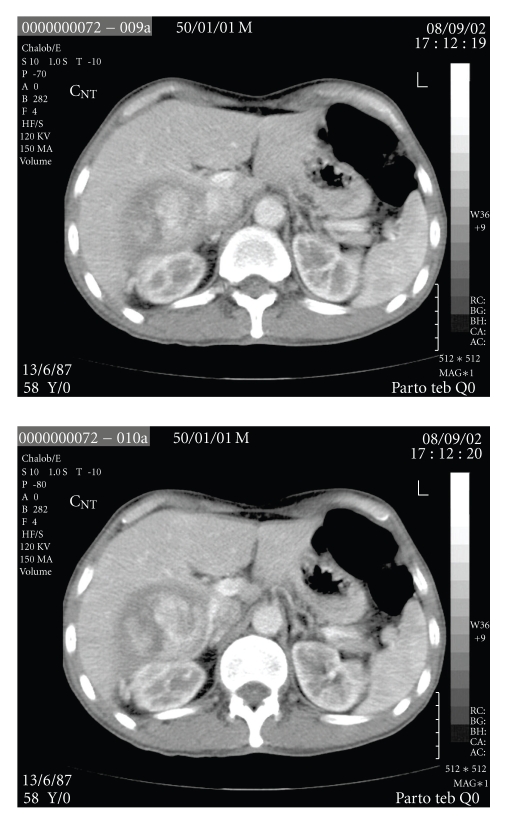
CT scan of the patient revealing the subhepatic mass.
